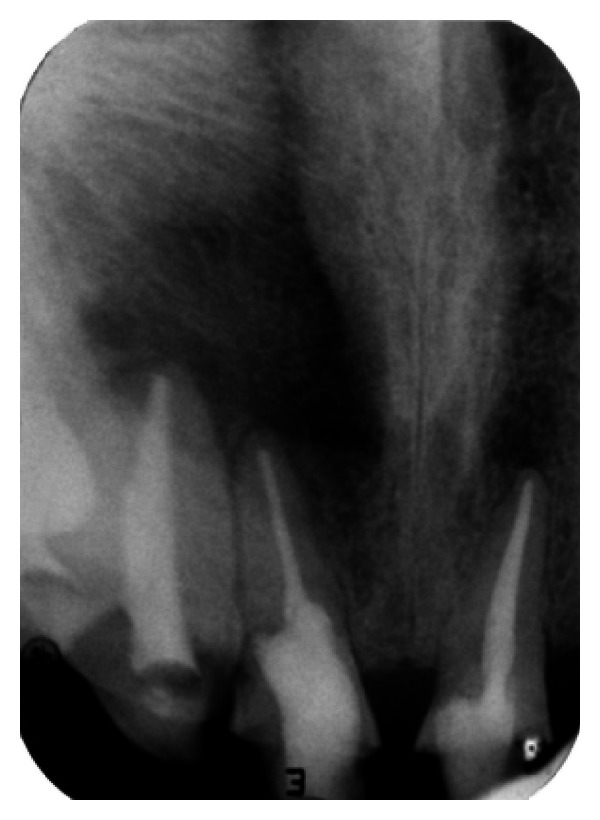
12-month followup IOPA radiograph.
